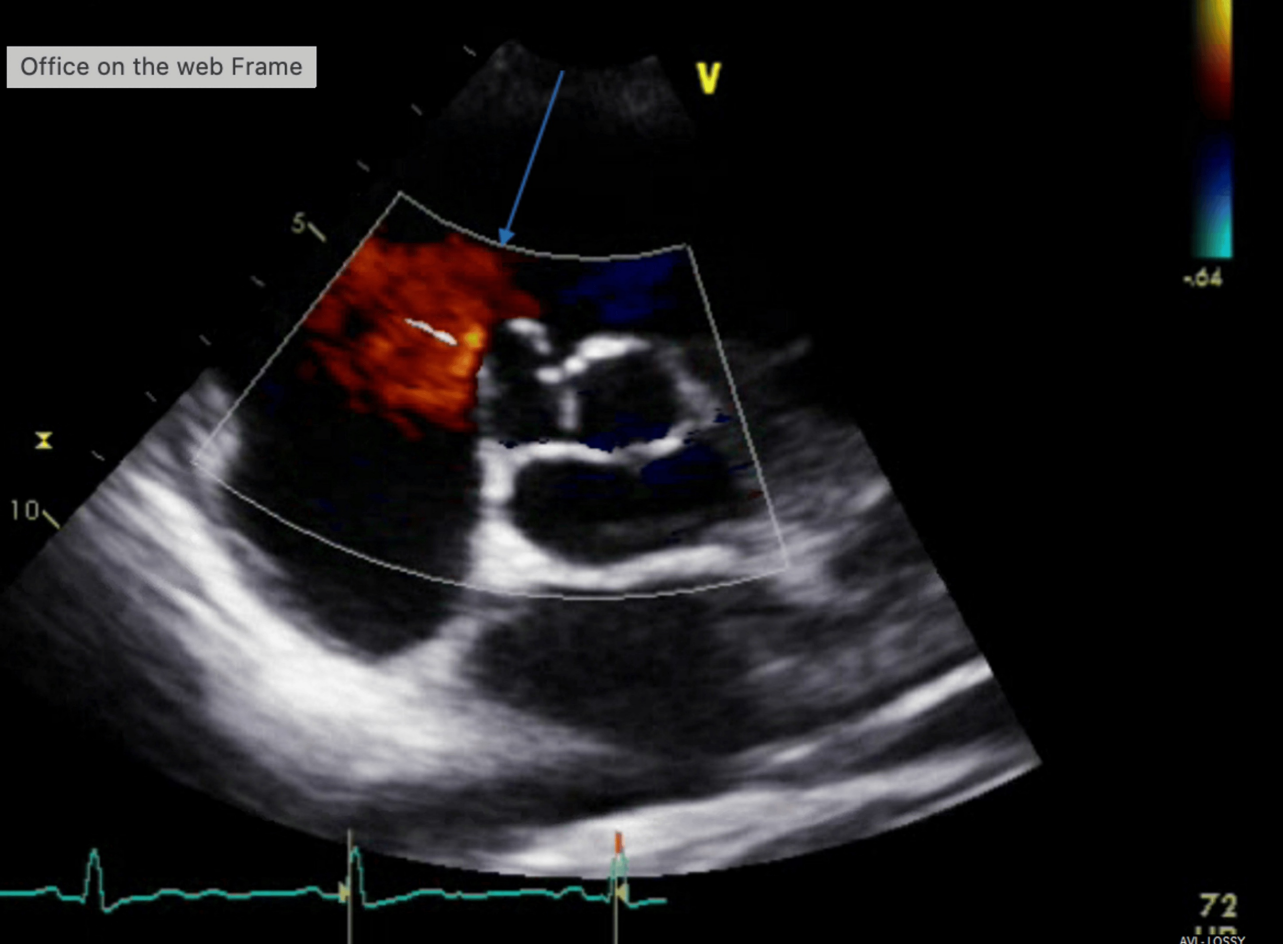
Short axis view at the level of the aortic valve from transthoracic echocardiography There is color Doppler flow overlying, displayed in red. The color flow is not crossing over from the right atrium/right ventricle into the sinus of Valsalva aneurysm, demonstrating a non-ruptured aneurysm. The blue arrow indicates the sinus of Valsalva aneurysm involving the right coronary cusp, measuring 11 mm (width) x 10 mm (depth). The image was taken during end diastole of the cardiac cycle.
